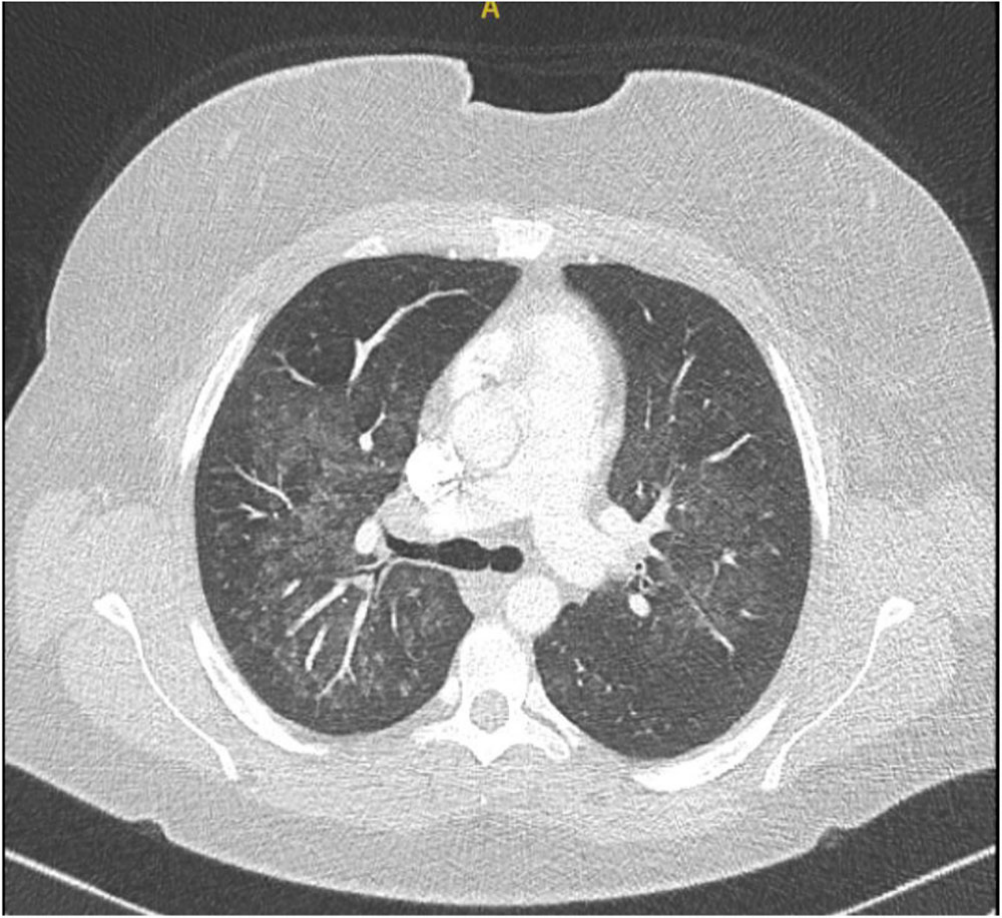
Chest CT for patient A showing ground glass shadowing at the level of the tracheal bifurcation.
